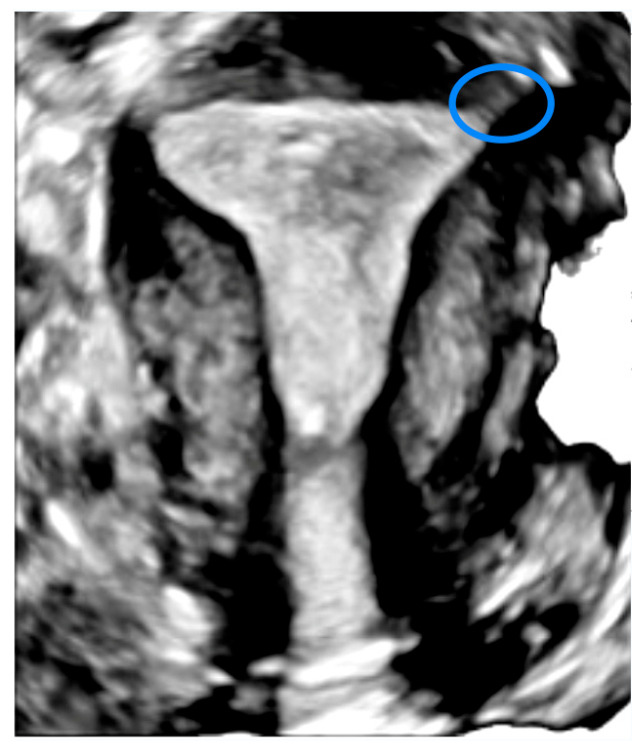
The interstitial segment on a 3D ultrasound image of the uterus—SIEOG, [46].
